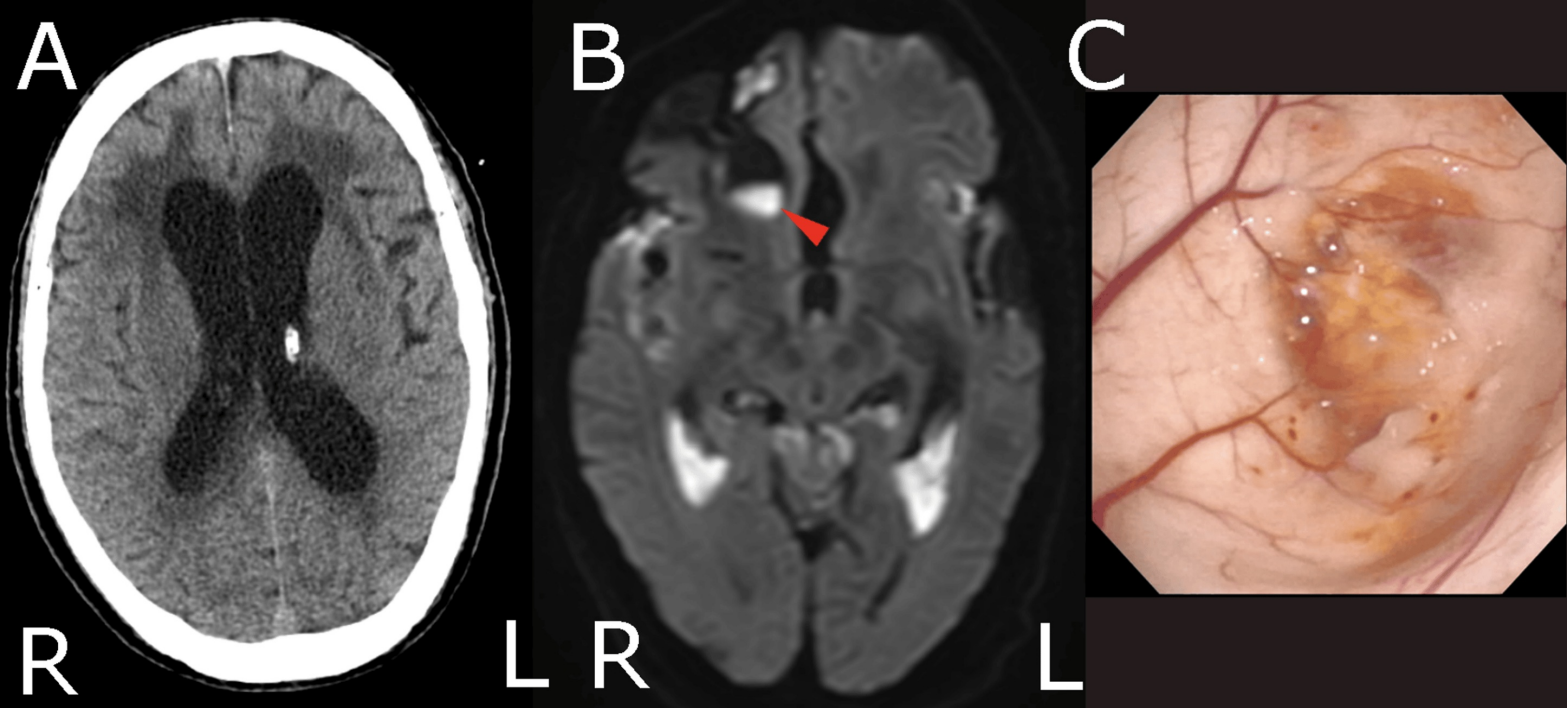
Imaging findings after admission and intraoperative endoscopic observations. A: Axial CT scan following ventricular drainage. B: Axial DWI image obtained on the fifth day of hospitalization. The red arrow indicates a new high-signal area in the right anterior horn of the lateral ventricle. C: Endoscopic observation of the right anterior horn of the lateral ventricle. The ependymal wall was partially defective, and an abscess invading the ventricle was observed through the ependymal vessels. DWI: diffusion-weighted imaging
